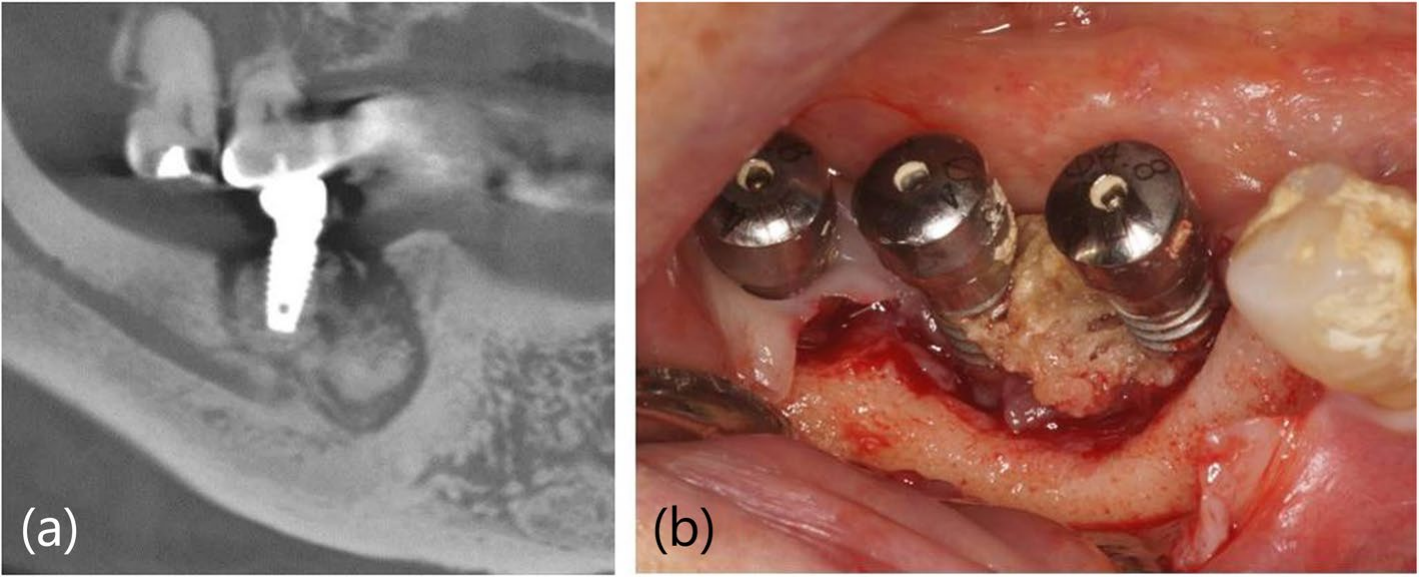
Necrotic bone sequestrated as a block including the implant in en bloc type. a Computed tomography image. b Clinical image
